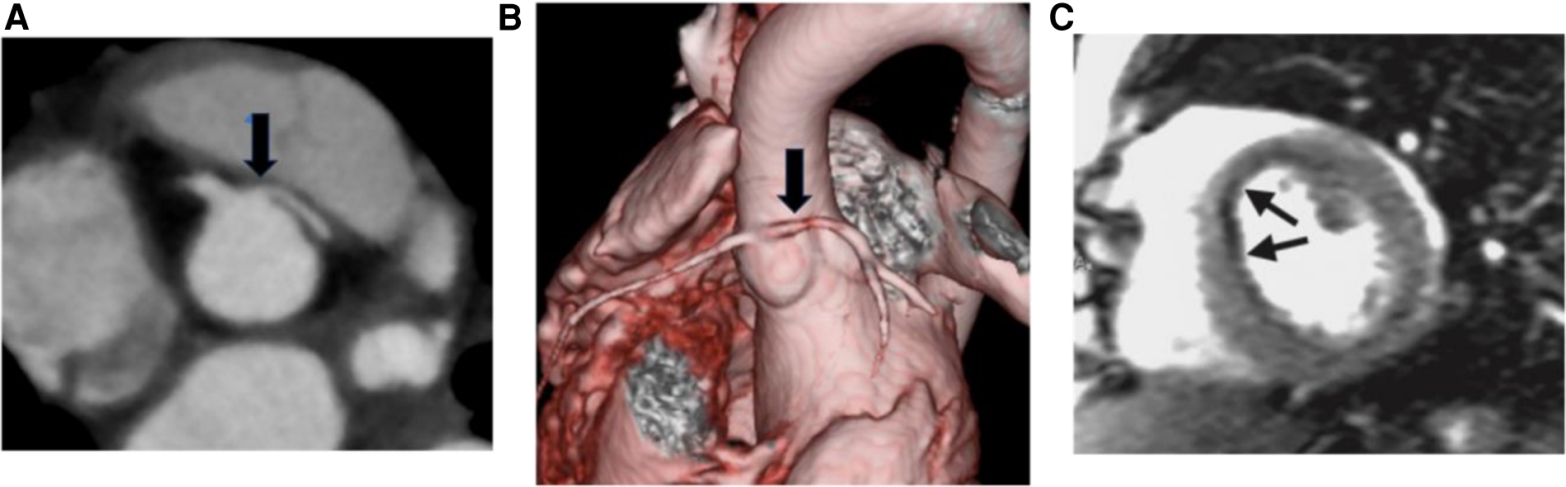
(A) Axial CTA image showing the anomalous aortic origin of the left coronary artery (L-AAOCA) (B) 3D reconstruction depicting the L-AAOCA. (C) Dobutamine stress cardiac MRI perfusion sequence showing a subendocardial perfusion defect in the anterior interventricular septum.
